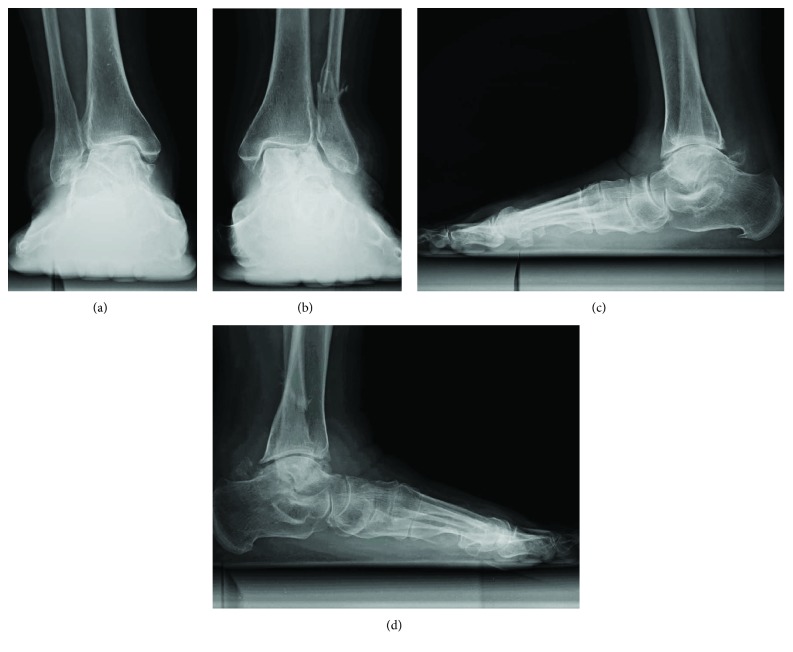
Plain radiographs obtained at the first visit. Anteroposterior weight-bearing radiograph of the right ankle (a) and left ankle (b) showing valgus talar tilt with joint space narrowing and collapse of the lateral talar dome. The left ankle was also accompanied by fracture of the distal fibula (b). Lateral weight-bearing view of the right foot (c) and left foot (d) showing the severe arch collapse and increased radiodensity of the body of the talus.
